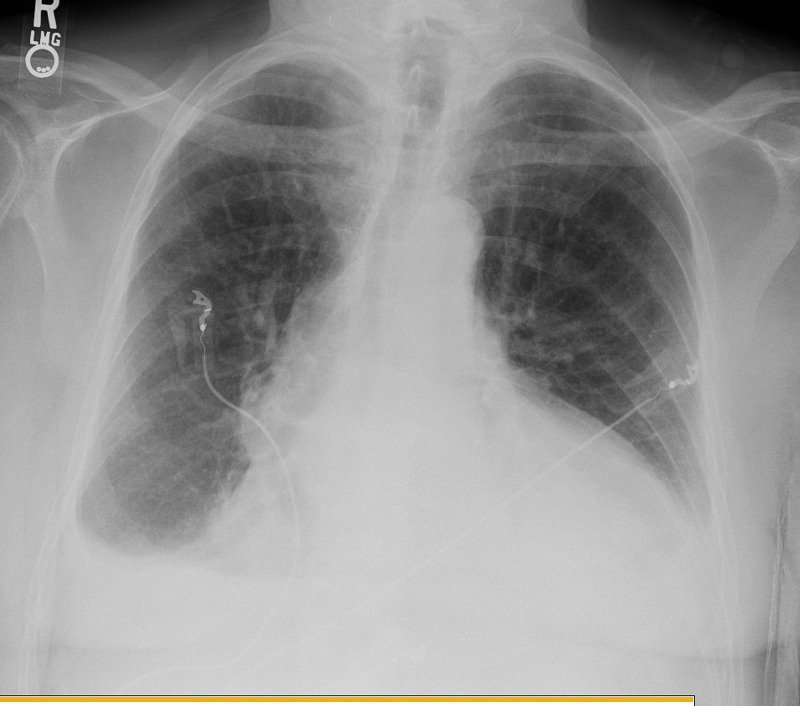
Chest radiograph remarkable for enlarged cardiac silhouette, new bibasilar airspace opacities, and small bilateral pleural effusion.
